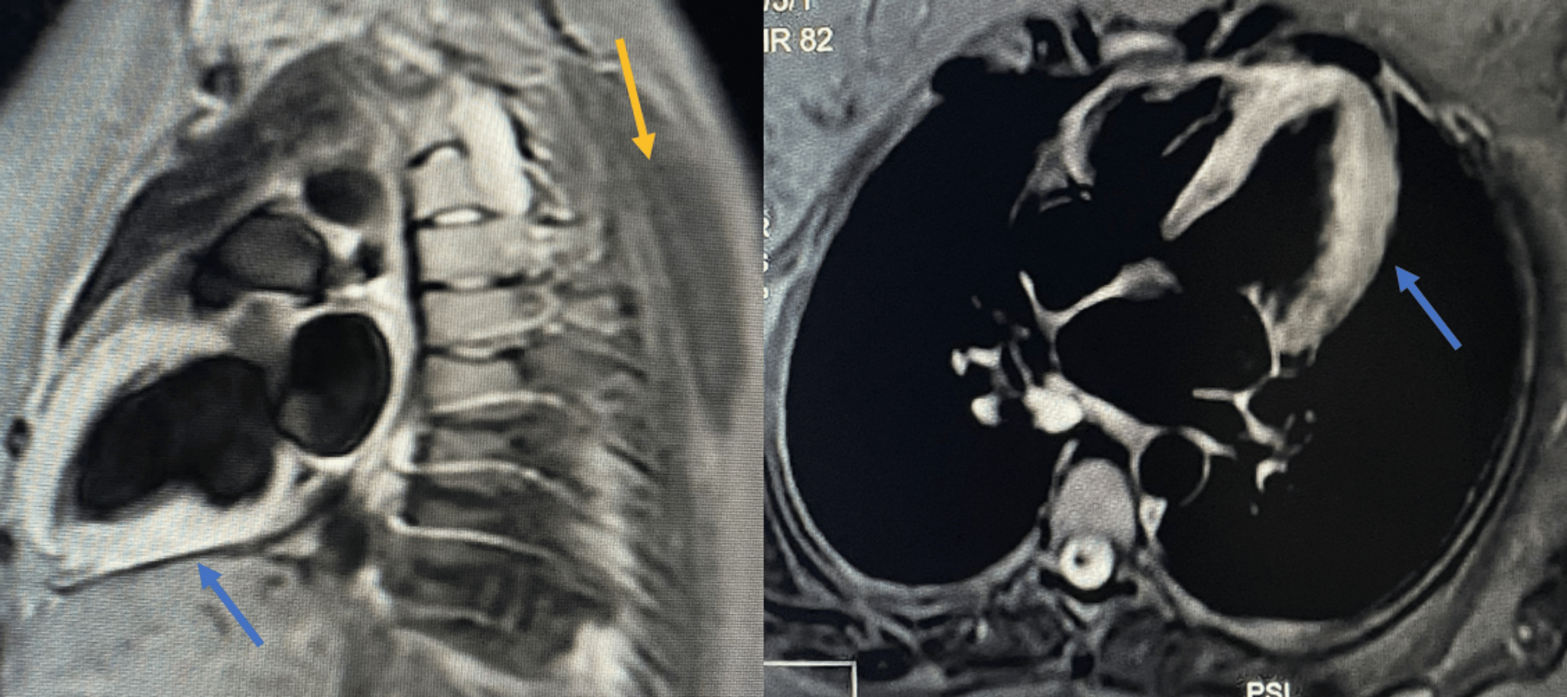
Cardiac MRI T2 short tau inversion recovery sequence in the two-chamber (left) and in the four-chamber (right) view: diffuse myocardial T2 hyperintensity compatible with myocardial edema, in comparison with adjacent dorsal skeletal muscles. Blue arrow: Myocardial hyperintensity. Yellow arrow: Dorsal skeletel muscles.
